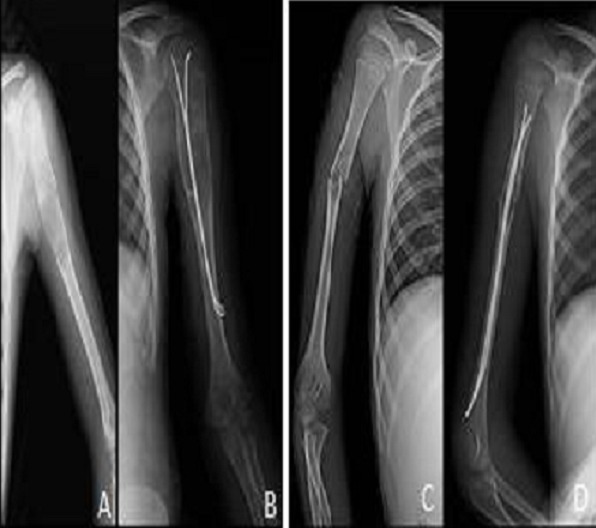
Radiography (A) showing a lesion: osteolytic multiloculated diaphyseal humeral very limited. The child was given a plug-curettage (B). Images C and D showing a lesion: osteolytic multiloculated diaphyseal humeral fracture very limited. The child received a pinning (D)
